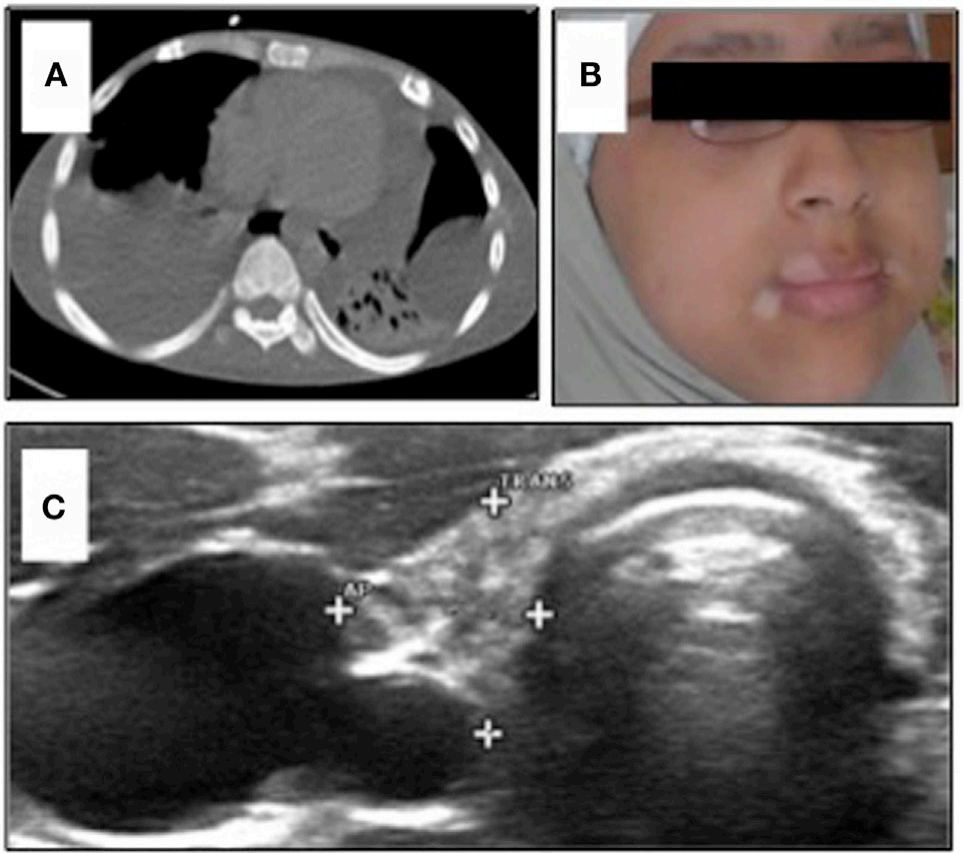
(A) Chest CT-scan of Patient 1 showing severe polyserositis with pleural and pericardial effusions; (B) patient 2 with progressive perioral vitiligo without other abnormalities in her face; and (C) ultrasound of the thyroid gland in Patient 2 showing a hypotrophic thyroid gland with 2.5 ml volume and several nodules <5 mm.
